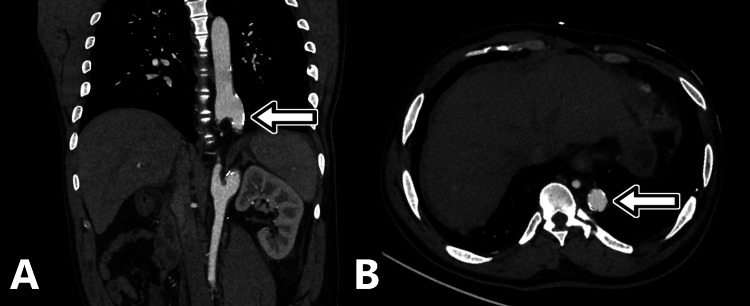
Coronal and axial CT angiography. The coronal projection (A) demonstrates both sites of bifurcation at the inferior descending aorta and superior abdominal aorta. The patent trunk (arrow) is ectatic with atherosclerotic calcifications.
